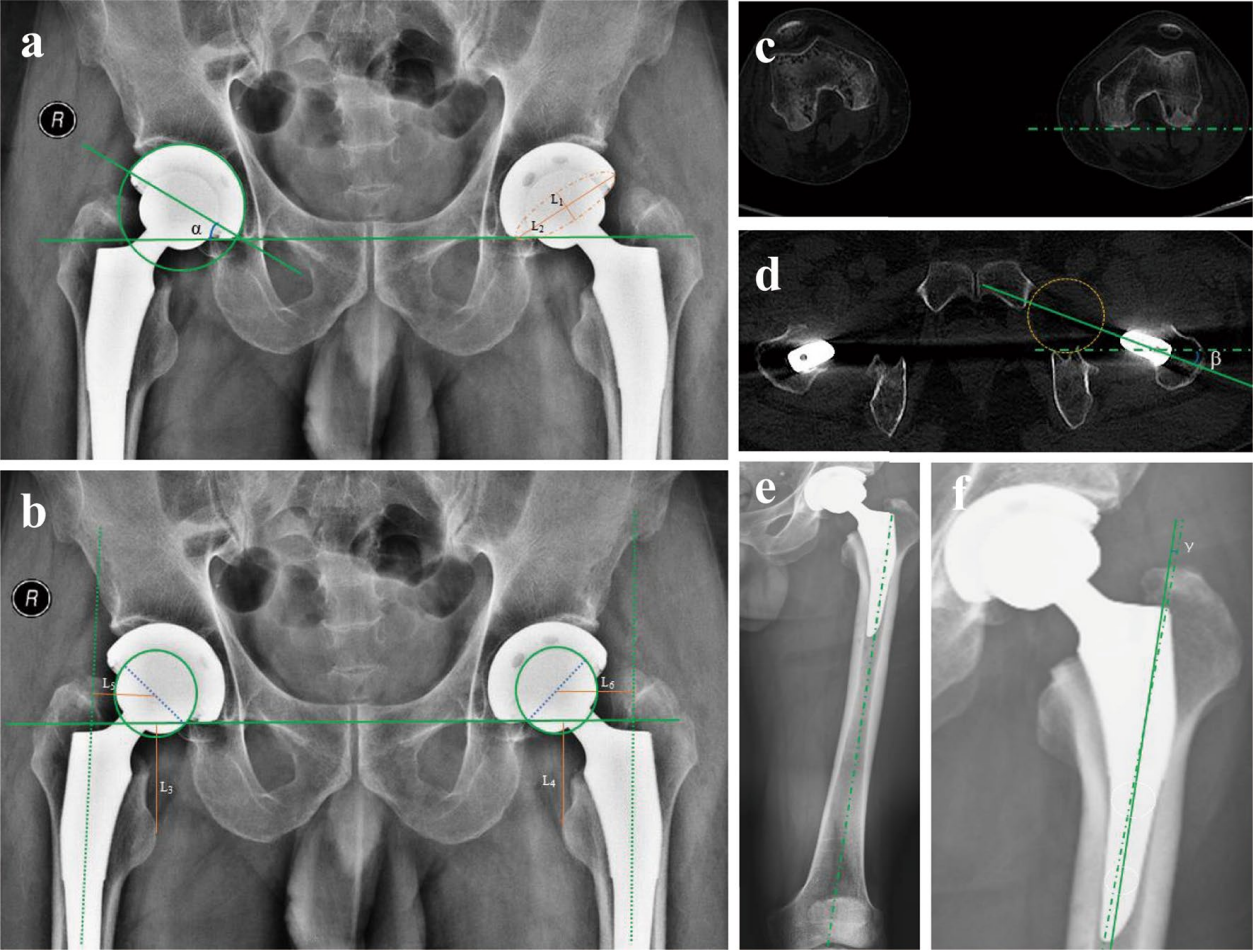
Radiographic measurements. (a) The methods of measuring the acetabular inclination angle (α) and acetabular anteversion angle on an AP radiograph, the long and short axes of the ellipse are used to calculate the acetabular anteversion angle, which is arcsin (L1/L2). (b) A line was drawn perpendicular to the inter-teardrop line and passed through the tip of the lesser trochanter on both sides (L3, L4). LLD was defined as the difference in distance between L3 and L4. Femoral offset was defined as the distance from the femoral head center to a line bisecting the long axis of the femur (L5, L6). (c, d) Femoral stem anteversion was defined as the angle (β) between the axis of the stem-neck and the posterior condylar axis of the femur. (e, f) Stem varus/valgus was measured as the angle (γ) between the anatomic axis of the femur and the axis of the femoral stem in the coronal plane. AP, anteroposterior; LLD, leg length discrepancy.
